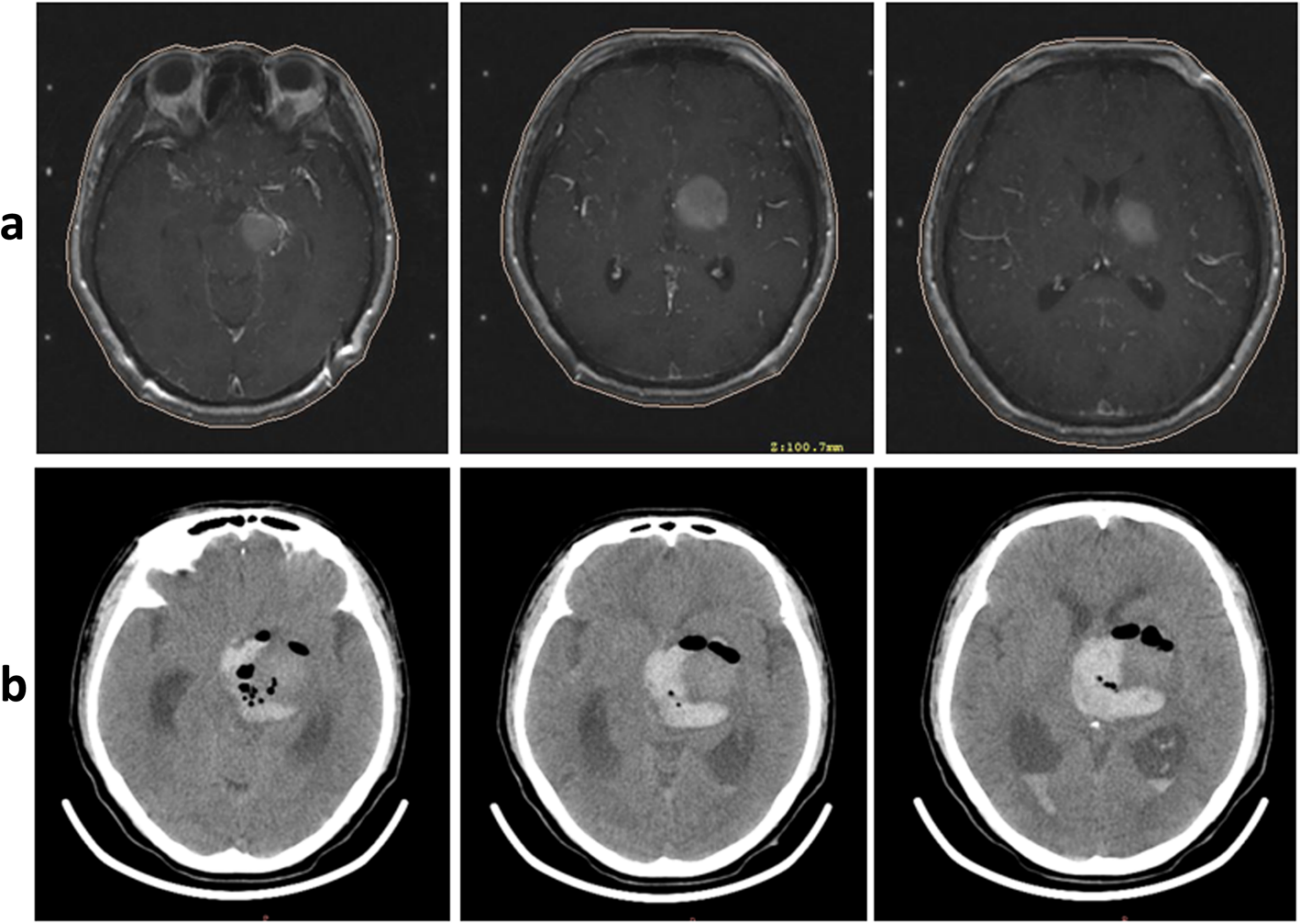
a Stereotactic postcontrast MR imaging scan demonstrated a left thalamoganglionic mass. b Postoperative CT scan showed intraparenchymal hemorrhage with intraventricular extension resulting from a prothrombin time longer than 15 s
